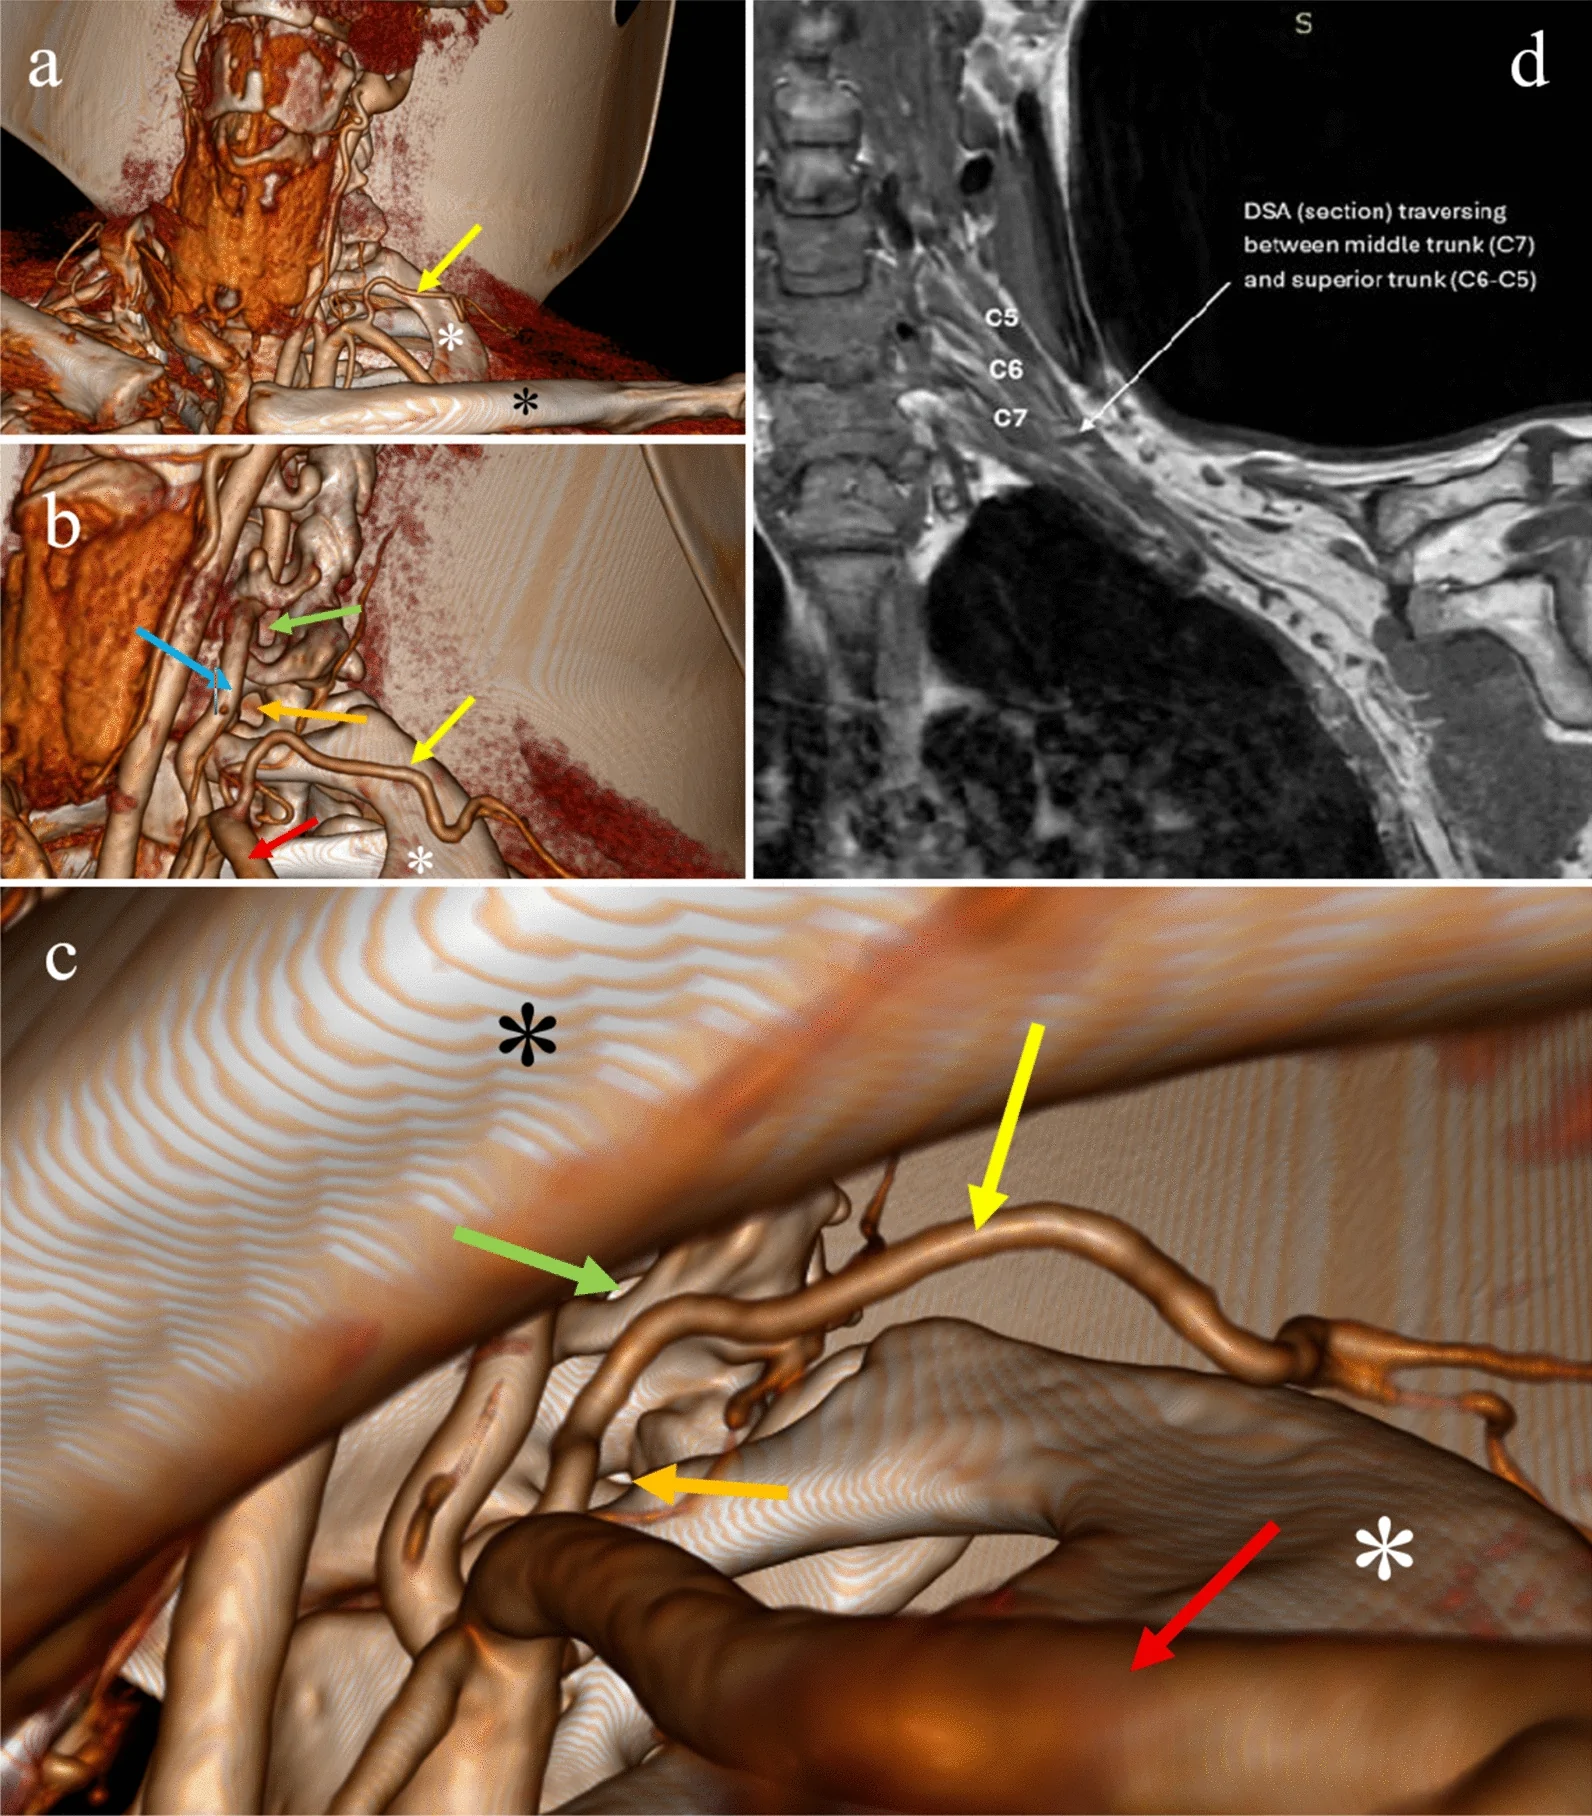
3D reconstruction of computed tomography angiogram a–c acquired after the injections showing the large, tortuous prominent DSA. a, b supraclavicular views. c infraclavicular view through the costoclavicular space. The MRI was analyzed retrograde after the injections, and the DSA relationship to the plexus could be appreciated (d). Yellow arrow: DSA. Red arrow: subclavian artery. Green arrow: C6–C7 foramen. Orange arrow: C7–T1 foramen. Blue arrow: vertebral artery. White asterisk: first rib. Black asterisk: clavicle
